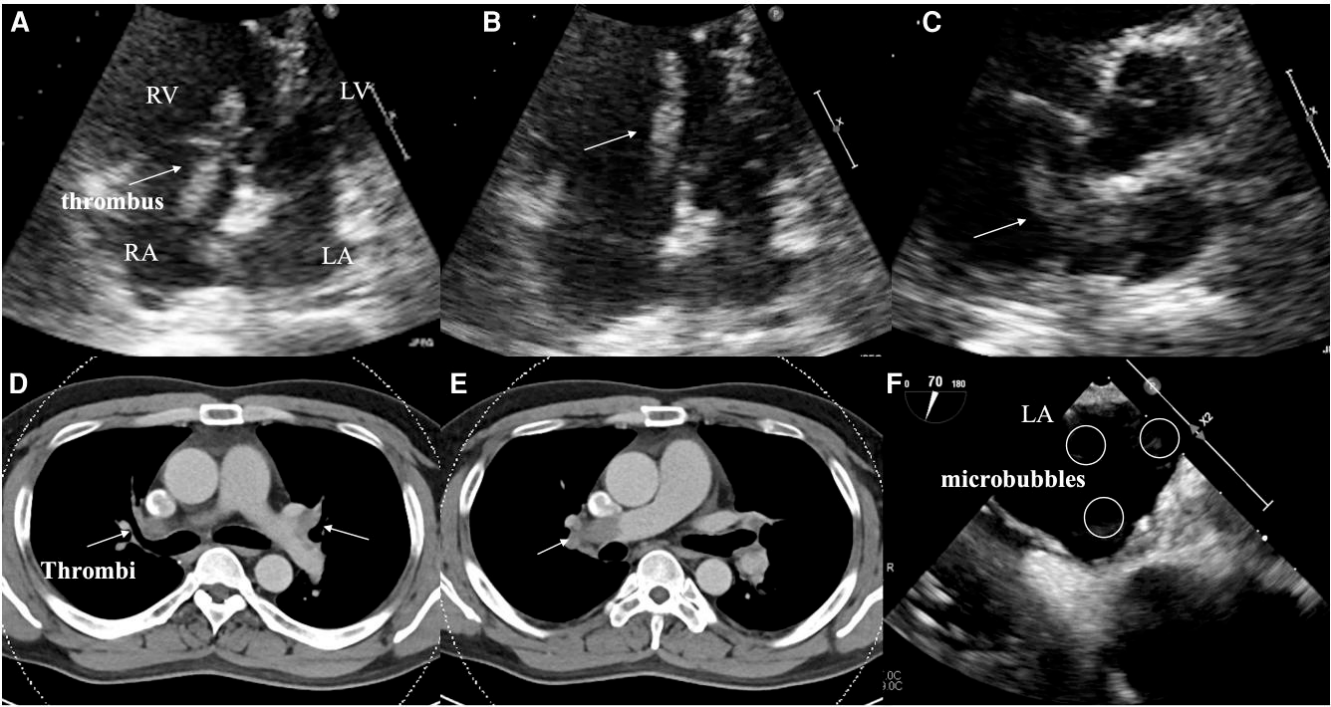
(*A–C*) A mobile, thread-like structure, approximately 4 cm in length, was observed within both atria. During diastole, the structure extended beyond the atrioventricular valves into the ventricles. It was trapped within a patent foramen ovale and positioned between the atria. (*D–E*) Thrombi were identified in both pulmonary arteries on contrast-enhanced computed tomography (CT). (*F*) Transoesophageal echocardiography performed on Day 9 confirmed resolution of the atrial thrombus; however, a bubble test demonstrated grade 2 microbubble passage into the left atrium, even in the absence of a Valsalva manoeuvere.
